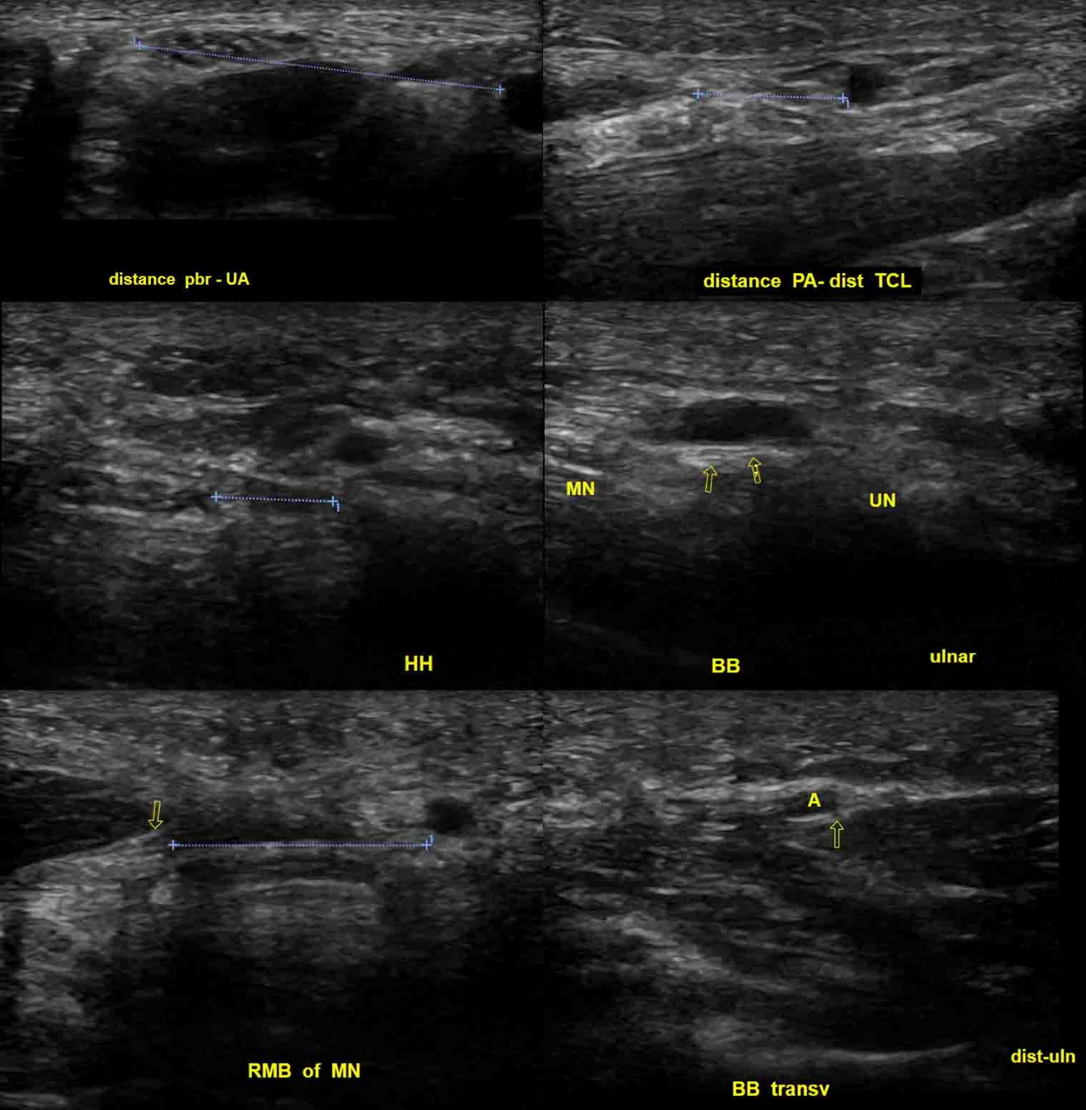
Ultrasound evaluation of the relevant neurovascular anatomy and measurement of important distances according to the sonographic carpal tunnel assessment protocol (Fig. 11). Distance between the PBMN and UA on a transverse image (upper left). Safe zone between the radial end of the ulnar artery and the median nerve at the height of the median nerve on a transverse image. Additionally, the hook of hamate can be visualized and the distance to the median nerve measured (middle left). Distance between the RBMN (arrow) and MN on a transverse image (lower left). Distance between the distal end of the TCR and UA/superficial arterial arch on a longitudinal image (upper right). Visualization of the BB longitudinally (two arrows) on a longitudinally-oblique image just underneath the UA/superficial palmar arch (middle right). Visualization of the BB transversely (arrow) just next to the UA on a longitudinally-oblique image (lower right)
